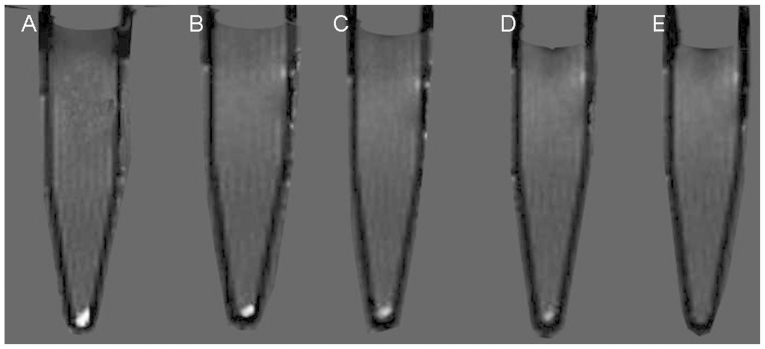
Tag persistence of BMSCs following labeling. As cell proliferation occurred, the T1-weighted image signal intensity of the BMSCs cells gradually decreased. (A-D) First, second, third and fourth cellular generation and (E) unlabeled cells, respectively. BMSC, bone marrow stromal cell.
